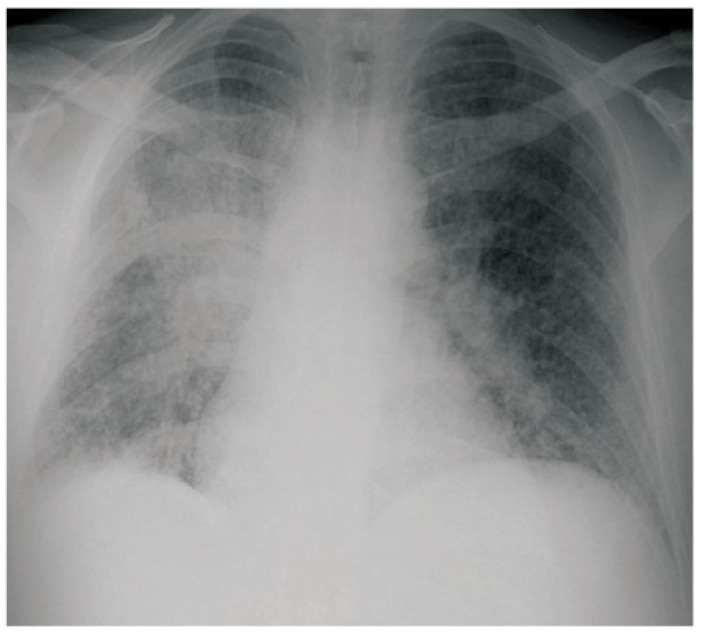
Pulmonary blastomycosis chest X-ray image. Chest X-ray seen in a patient with pulmonary blastomycosis demonstrating a right lower lobe consolidation and bilateral military nodules. Image sourced from Sarkar et al. under a creative commons license (CC BY 3.0) [56].
